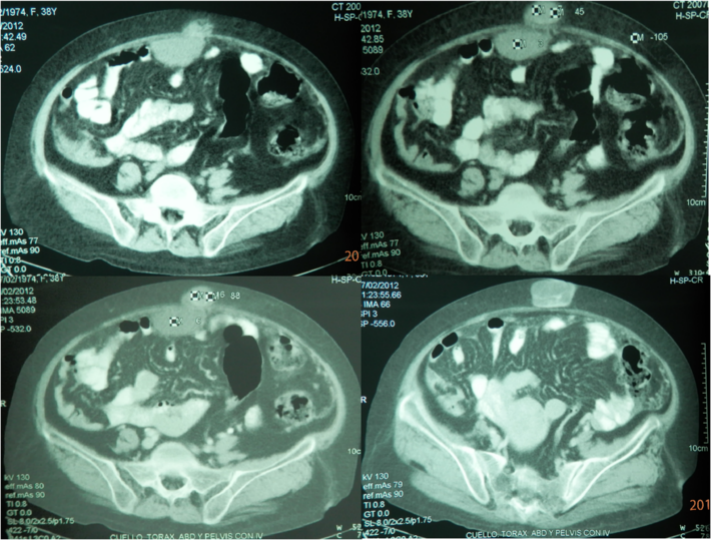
Abdominal computed tomography (CT) showed a bilobed image with protrusion through the umbilicus, with attenuation coefficients of 3, 7 and 45 HU; after administration of contrast enhancement they reached 6, 16 and 88 HU. Multiplanar reconstructions showed that its origin was intra-abdominal, adjacent to a loop of small intestine, and the possibility of a mesothelioma was considered
